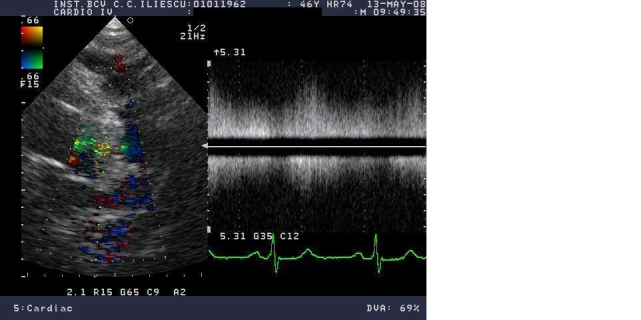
Patent ductus arteriosus. 2D and Doppler transthoracic echocardiography evaluation. In suprasternal section just below the subclavicular artery origin, the continuous flow of patent ductus arteriosus is seen, with maximum velocity of 5,3 m/s.
